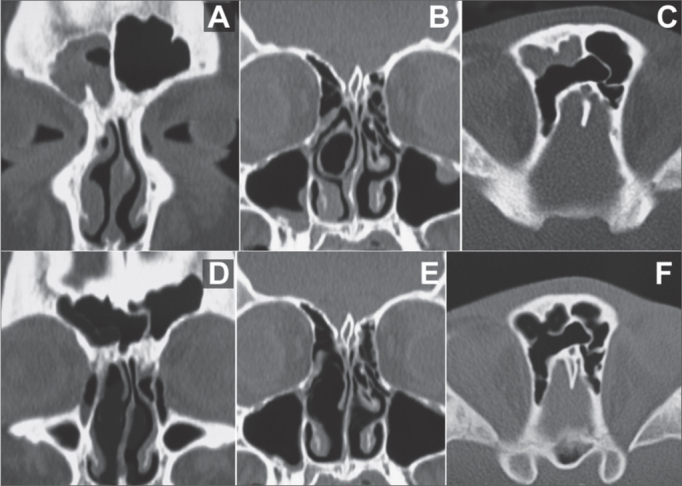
Pre and post-operative CT scan of a patient submitted to septoplasty, partial middle turbinectomy, right-side maxillary and frontal sinuplasty and conventional frontal sinusectomy on the right side. A: coronal cross-section pre-operative image showing right-side frontal sinus blurring. B: Coronal cross-section pre-operative image showing septal deviation, bullous right-side middle turbinate and images showing soft tissue on the floor of the right maxillary sinus. C: Axial, pre-operative view showing right frontal sinus blurring. Notice the presence of supraorbital ethmoidal cell, representing a challenge in the proper identification of the true frontal cavity. D: Post-operative exam (3 months after the procedure) in a coronal cross-section showing normal aeration of the right-side frontal cavity. E: Post-operative coronal cross-section showing the results from the partial right-side middle turbinectomy and the septoplasty. F: Axial cross-section of the post-operative exam showing normal aeration of the right-side frontal sinus. Notice the shunt created with the supraorbital ethmoidal cell through conventional frontal sinusectomy.
